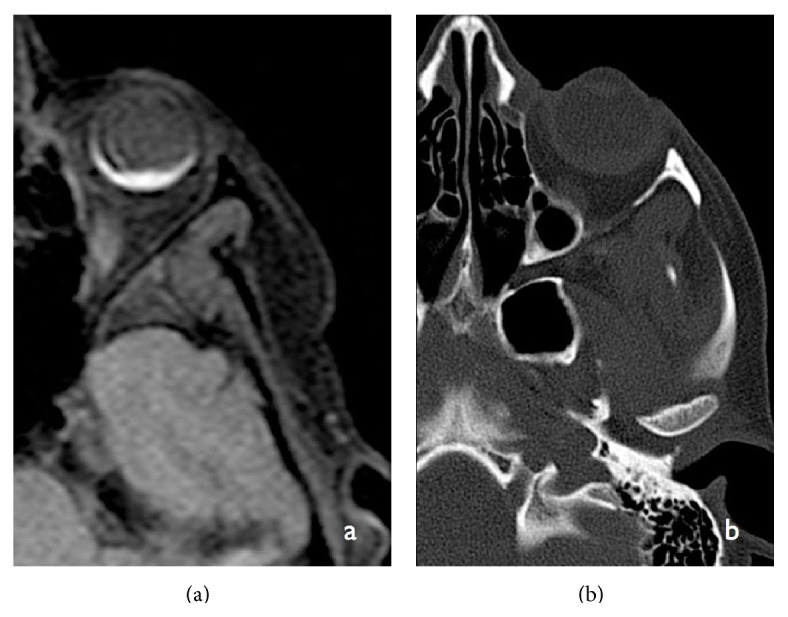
Axial T1-weighted image MRI (a) showing hyperintense lesion in the retinal choroid with a mild exophytic thickening of the uvea. Axial noncontrast enhanced CT section (b) in bone window projection showing the lesion to be of soft tissue densities without calcification.
